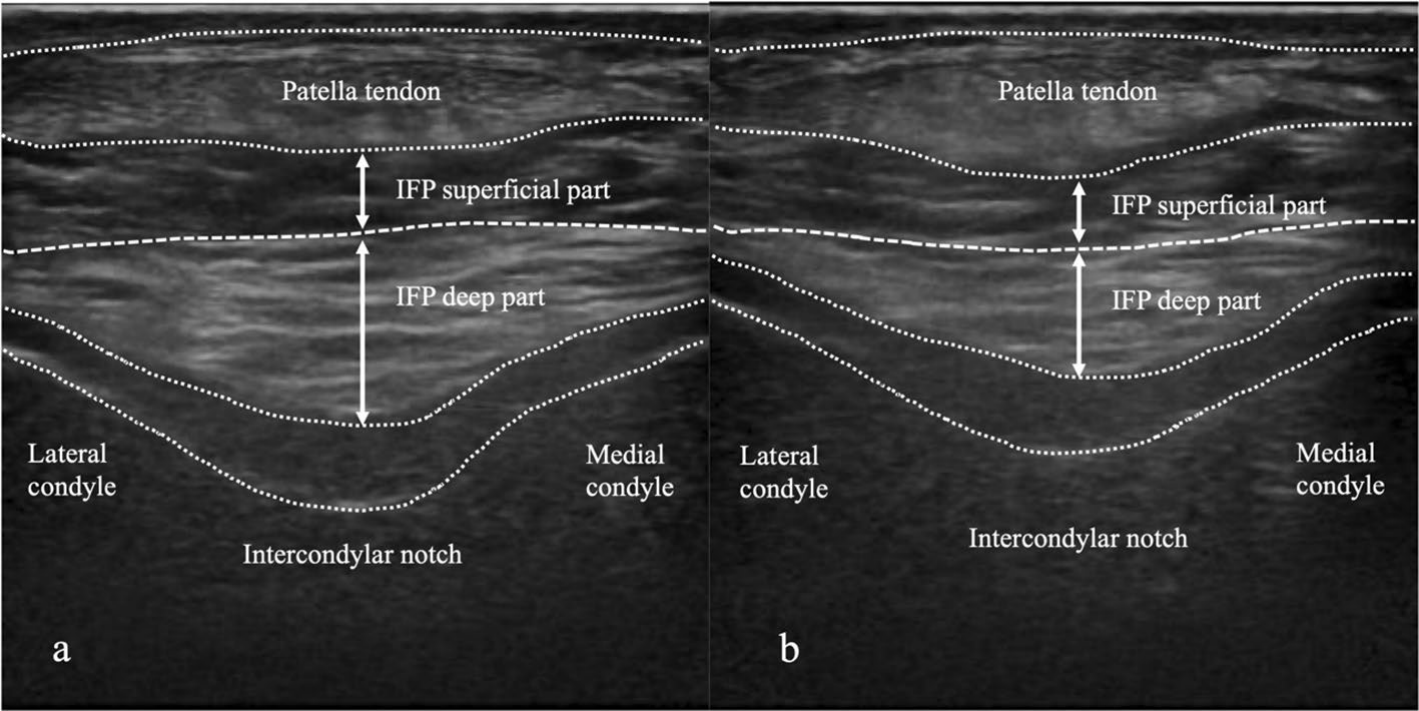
Ultrasonography image. The thickness of the superficial and deep part of the IFP was measured at 30° of flexion (a) and 0° of extension (b) of the knee joint. IFP, infrapatellar fat pad
